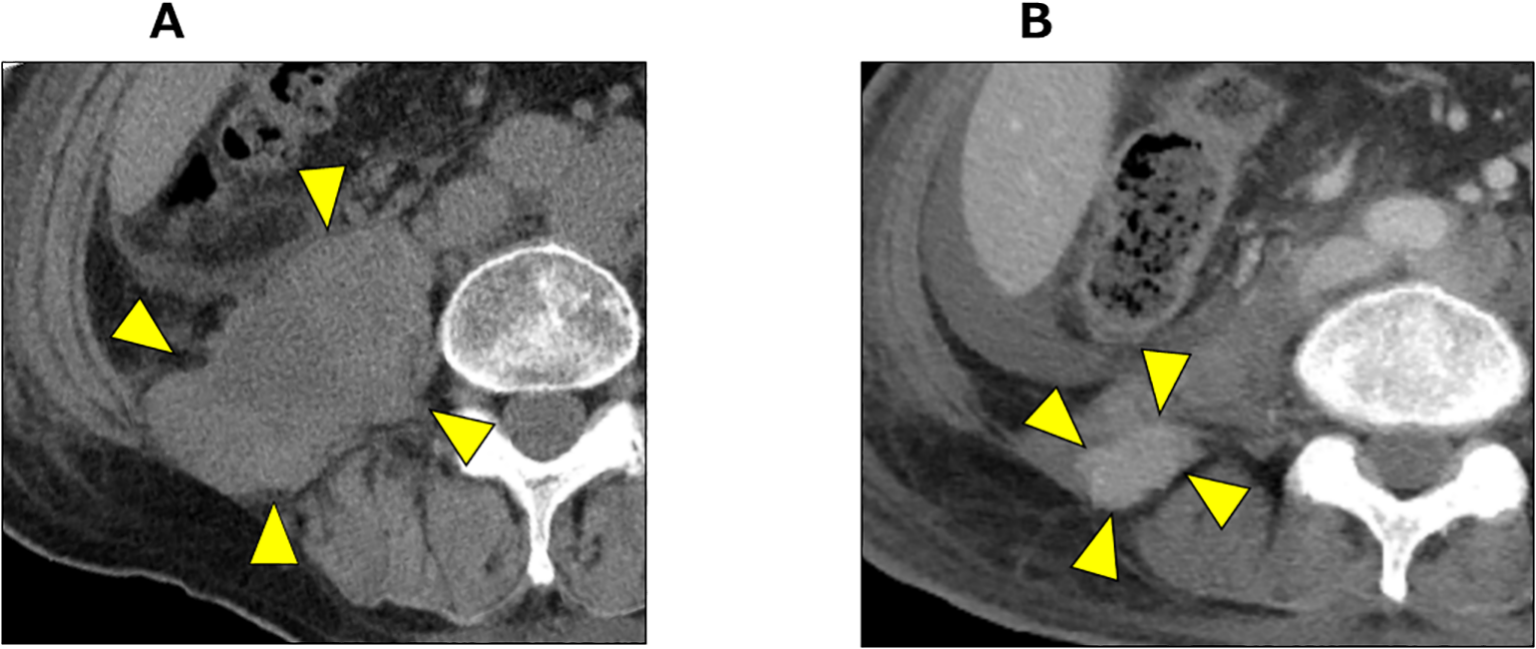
CT imaging before and after pembrolizumab administration. (A) CT imaging of retroperitoneal mass before pembrolizumab administration (arrow heads). (B) CT imaging 2 months after pembrolizumab administration. The retroperitoneal mass showed significant shrinkage (arrow heads).
